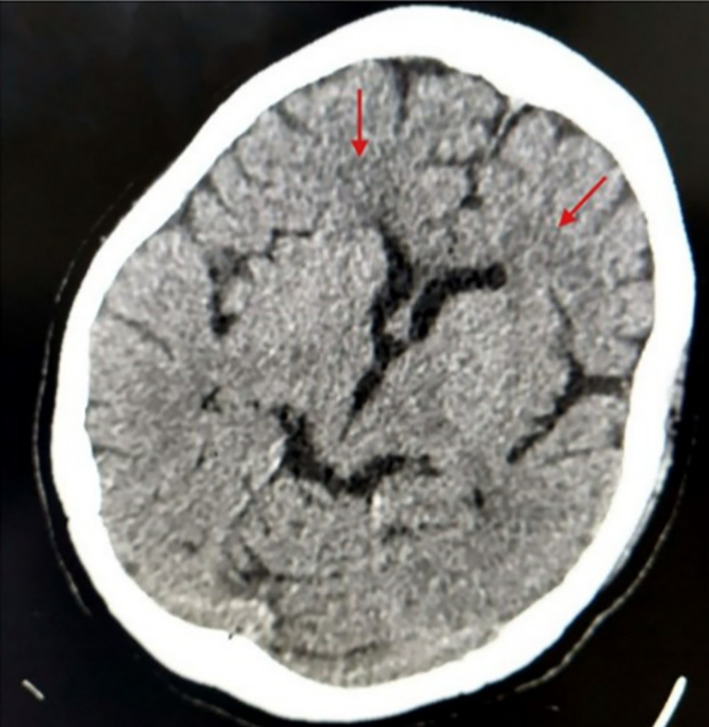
Computed tomography scan of the head showing white matter hypodensities (red arrow) in bilateral frontal lobes.
